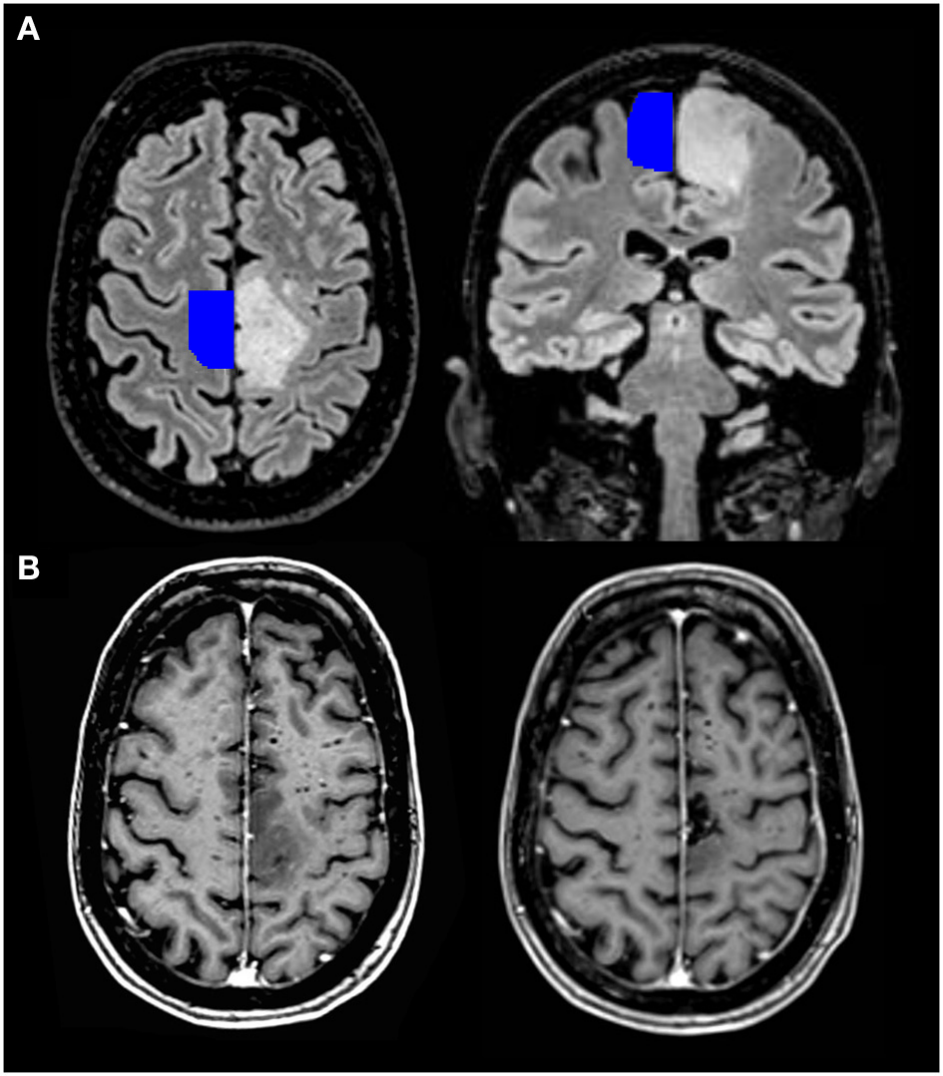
Pre- and post-operative MRI scan of a 64-year-old patient with a diffuse astrocytoma (WHO grade II) in the left SMA. (A) Transversal and coronal T2-weighted FLAIR images, with an SMA template projected on the healthy hemisphere. The latter is freely available and derived from a large meta-analysis describing the location of the sensorimotor areas (Mayka et al., 2006). (B) Transversal images after gadolinium contrast from the same patient before (left lower corner) and three months after the operation (right lower corner). She had a complete motor loss on the right side after the operation, which quickly recovered.
